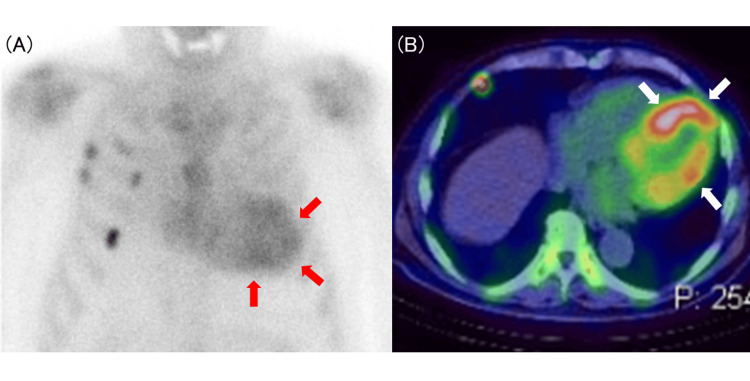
Nuclear imaging with technetium-99 m pyrophosphate (99mTc-PYP) scintigraphy. (A) 99mTc-PYP scintigraphy showed significant myocardial accumulation (Perugini grade 3) with a myocardial-to-lung ratio of 1.68 (planner anterior view). (B) Trans-axial view of a single-photon emission computed tomography (SPECT) image. Arrows indicate 99mTc-PYP uptake in the myocardium, with red arrows in (A) and white arrows in (B).
